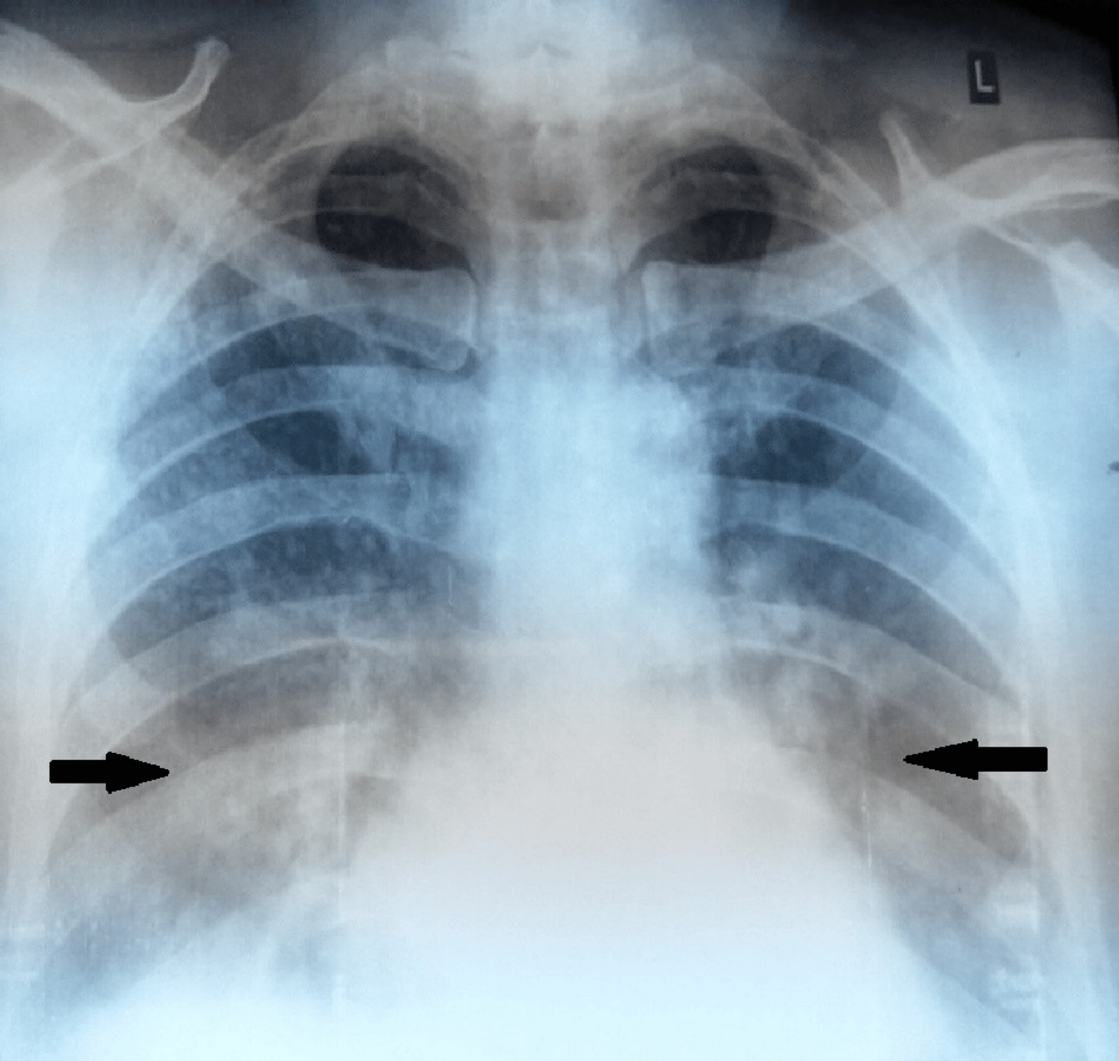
Chest X-ray of the patient, with arrows showing bilateral infiltrates.
